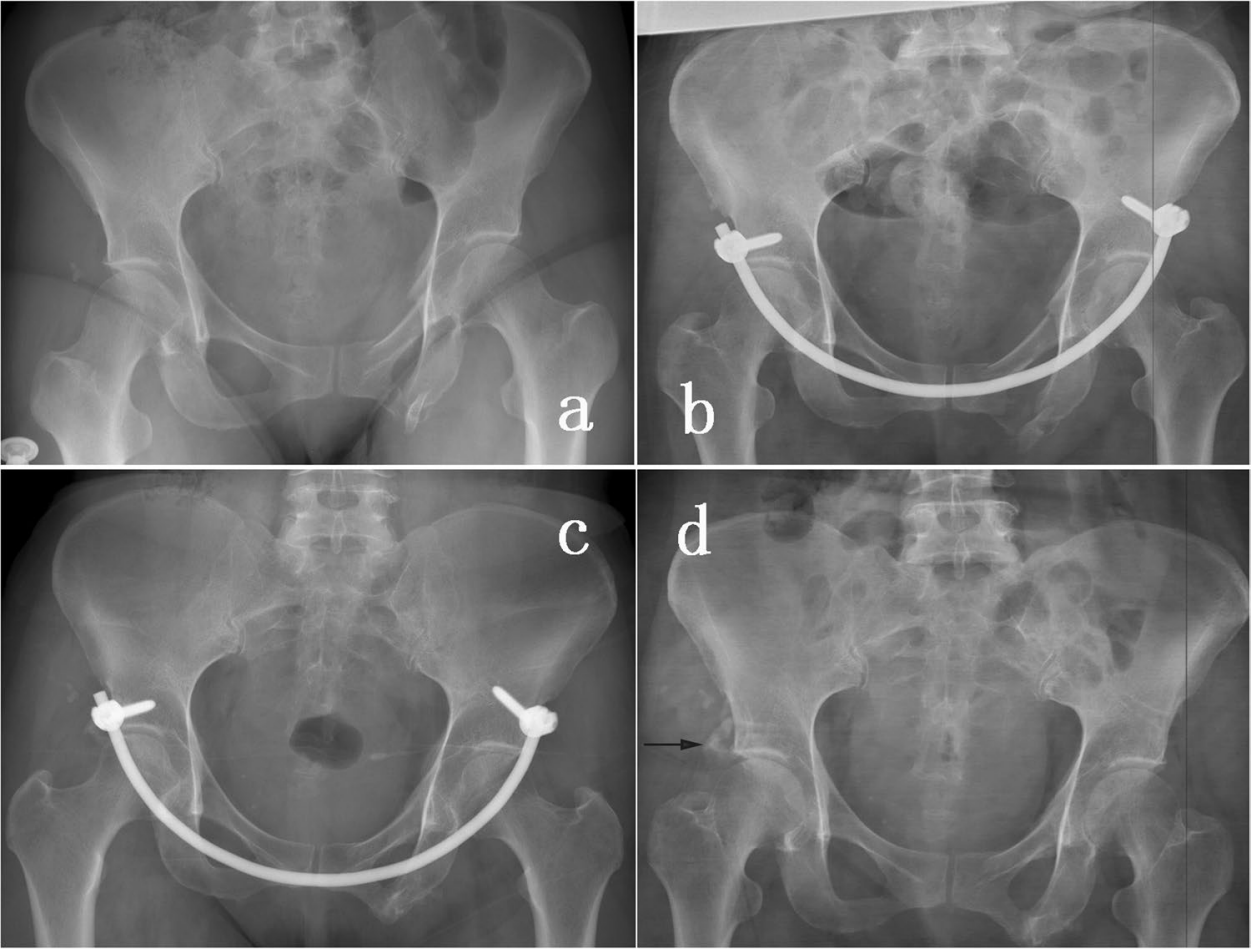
(a) AO/OTA 61-B2 pelvic ring injury. (b) Postoperative pelvic AP view. A “distraction force” was performed during locking the second pedicle screw end cap. (c) Six months follow-up AP view x-ray. (d) Pelvic AP view after removal of the INFIX. The black arrow indicates heterotopic ossification.
